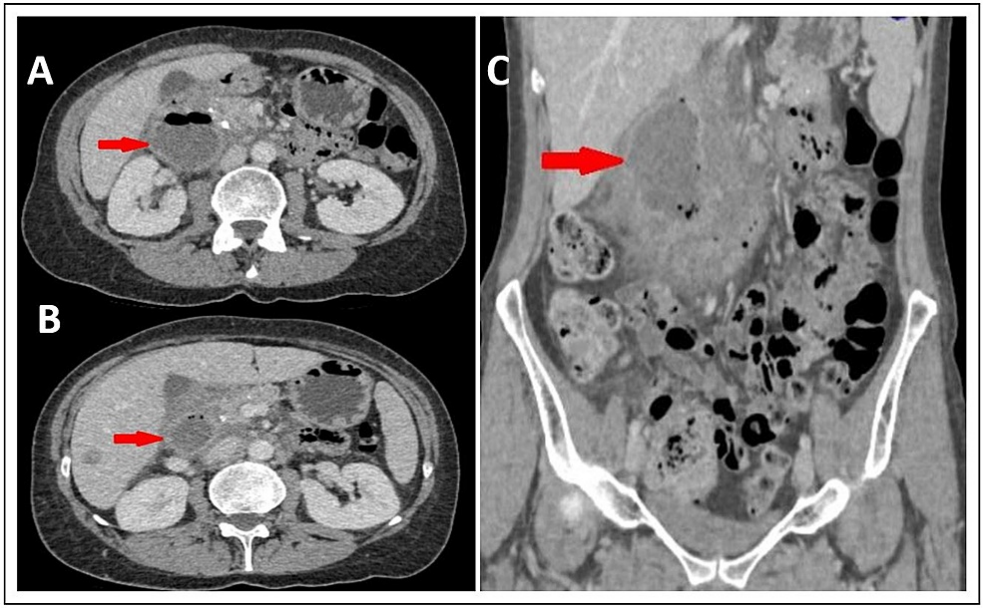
Preoperative CT scan Axial (A, B) and coronal (C) slides of the preoperative CT scan showing a D2 duodenal collection of 75 x 36 x 44 mm (red arrows)
